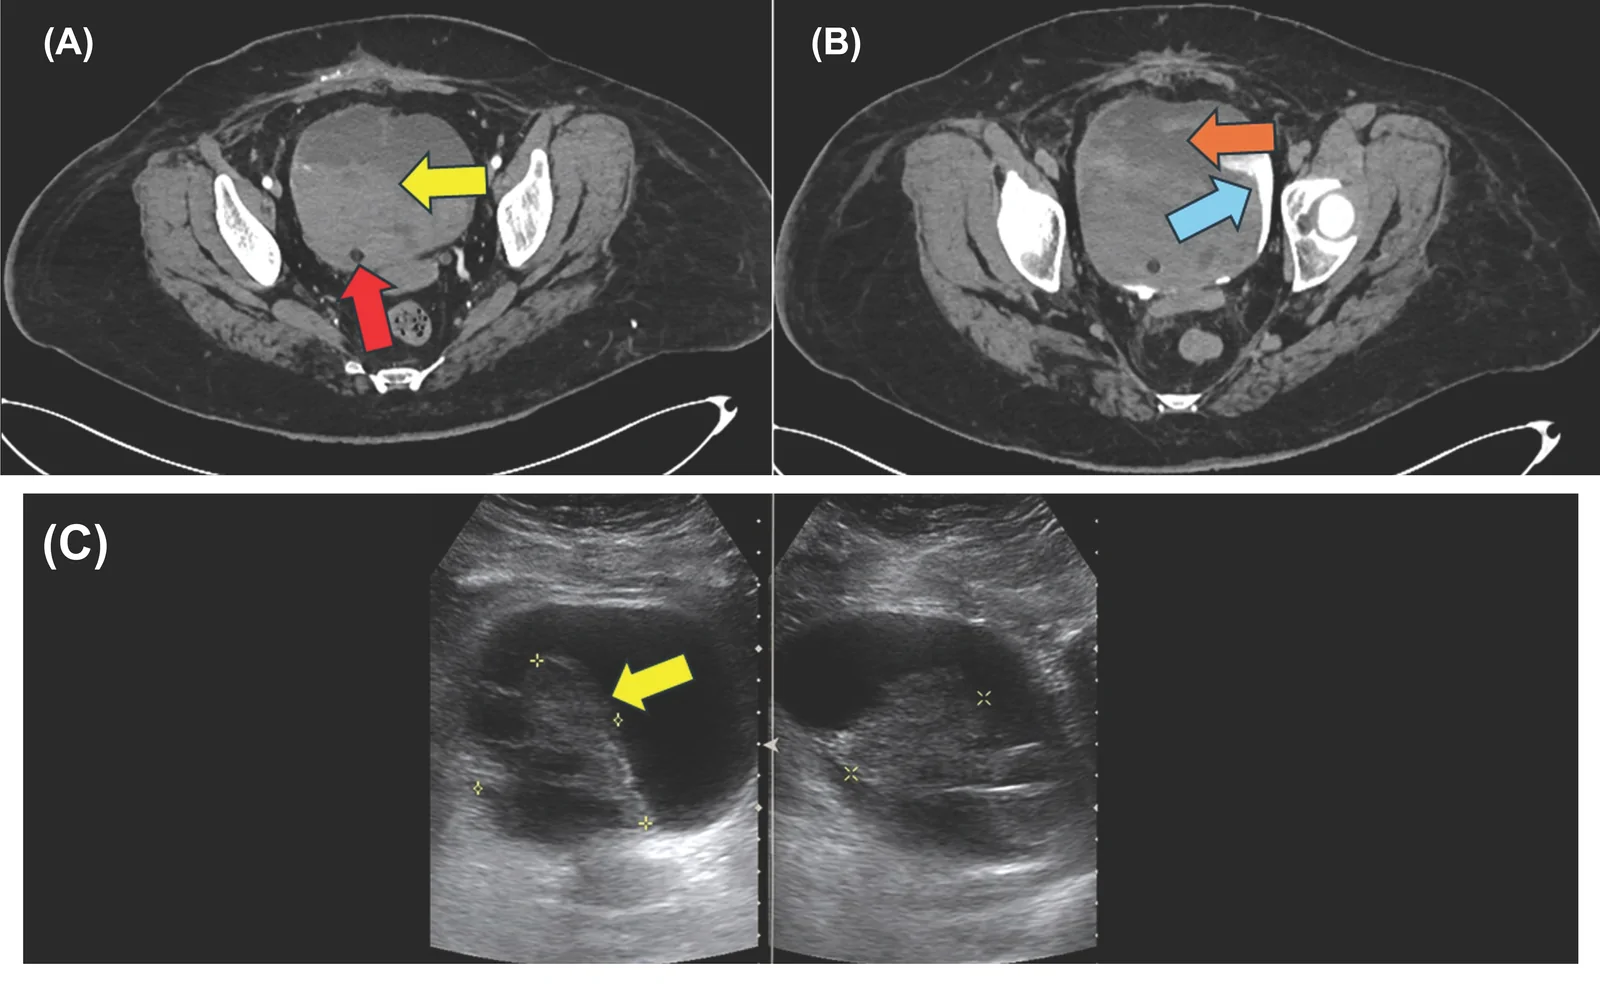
Bladder imaging CT and ultrasound (US). Non-contrast CT (A) and excretory-phase CT (B) show a distended bladder with a Foley catheter (red arrow), hyperdense clots ~48 HU (yellow arrow), and filling defects ~18 HU (orange arrow) compatible with hematic/granulomatous material. US (C) demonstrates an echogenic mobile intravesical mass causing incomplete emptying. Abbreviations: CT, computed tomography; US, ultrasound; HU, Hounsfield units.
